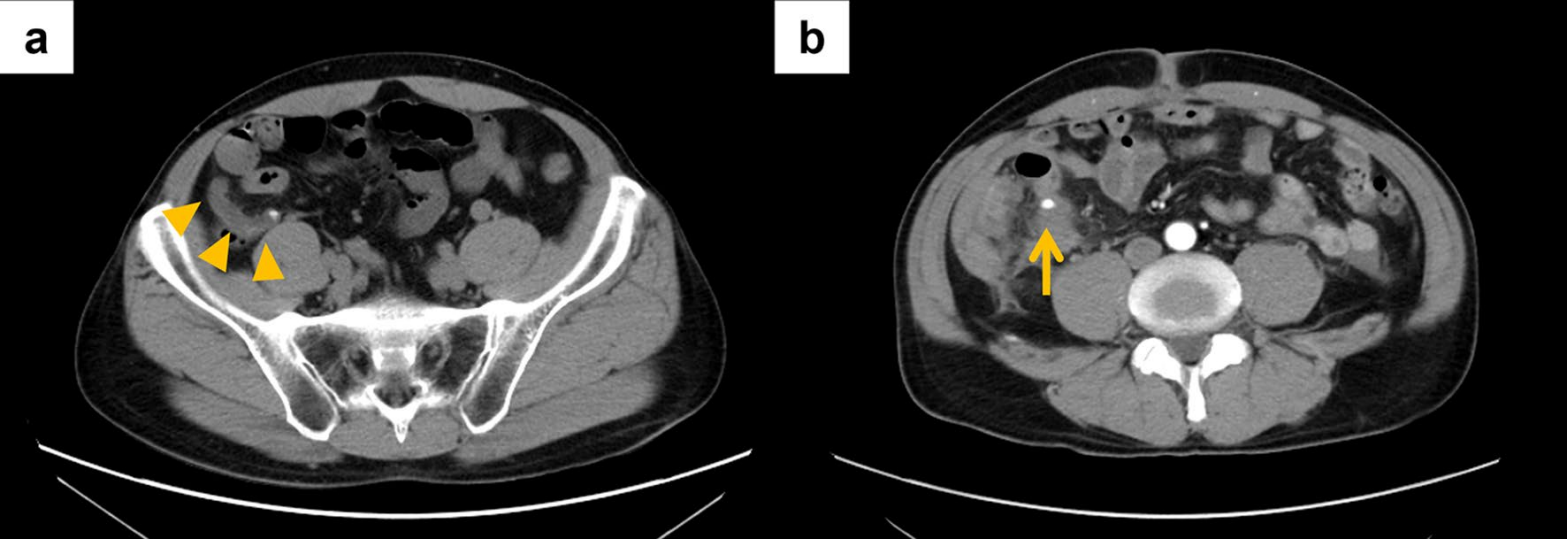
Abdominal contrast-enhanced CT findings. a At the first admission, a swollen appendix with a stercolith was observed at the root (arrowheads). b At the re-admission, a hematoma and a nodule suspected of being a pseudoaneurysm (arrow) were found in the ileocecal region
